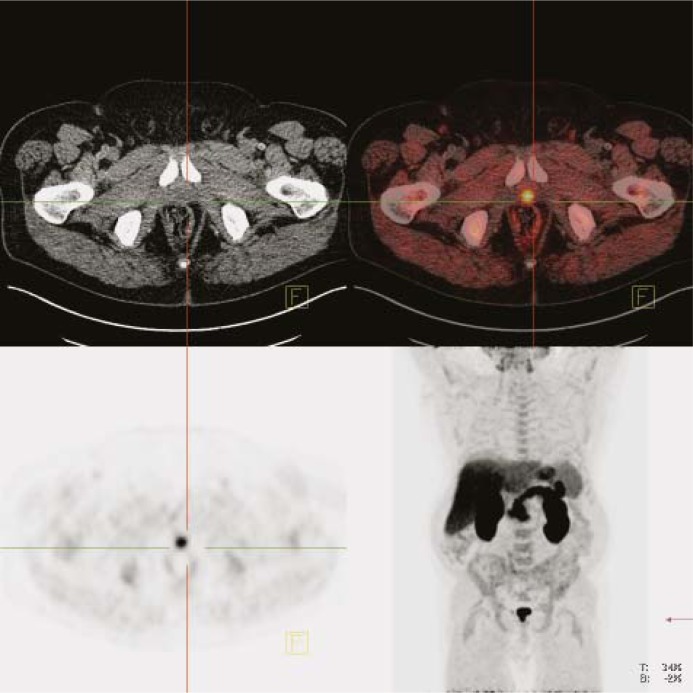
Relapse of prostate cancer: whole body (late images). Upper left panel: CT image. Upper right panel: fused PET/CT image. Lower left panel: PET image. Lower right panel: maximum intensity projection (MIP).
